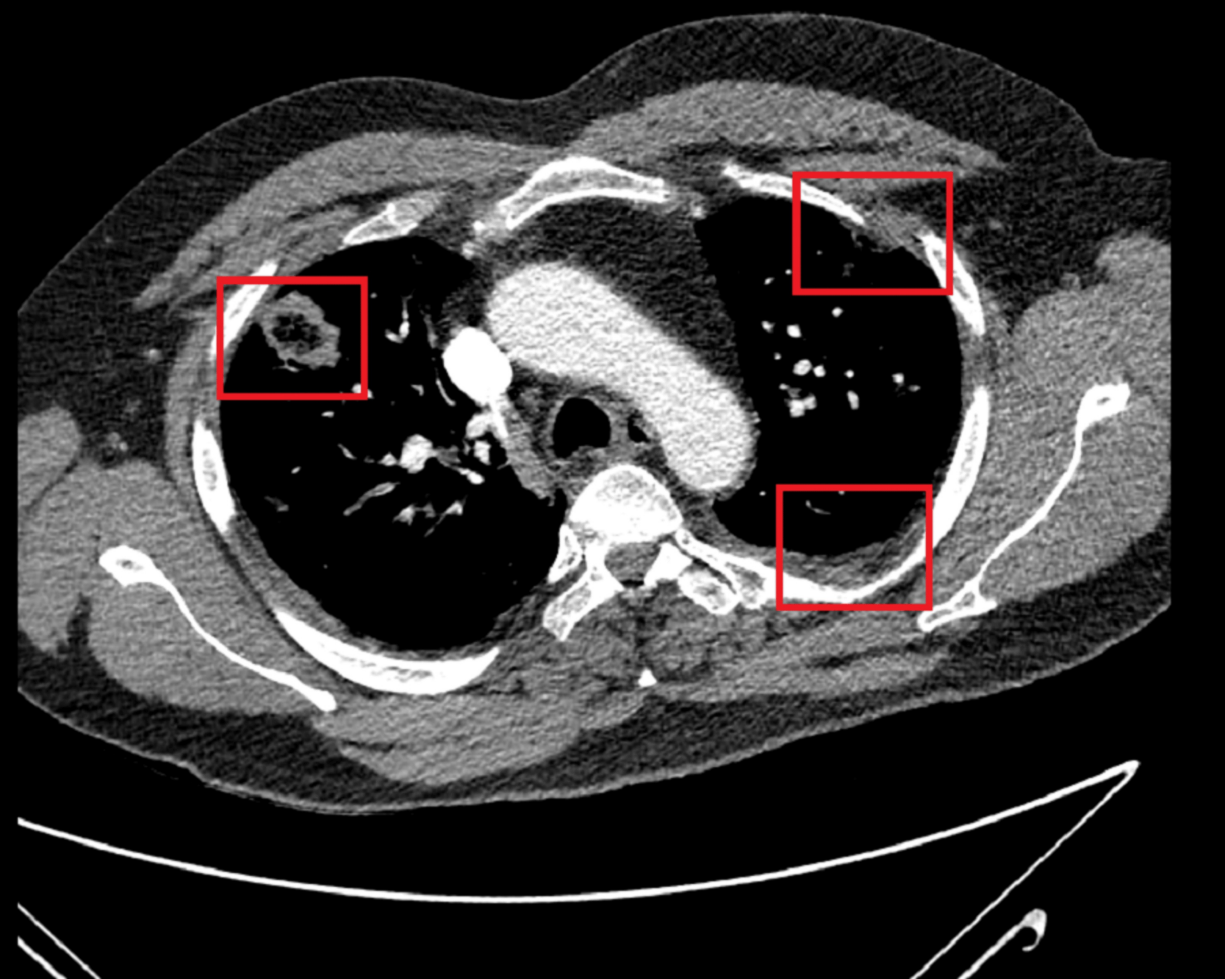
CT scan of the chest showing bilateral necrotizing pneumonia predominantly in the middle lung zones, with associated left-sided pleural effusion
